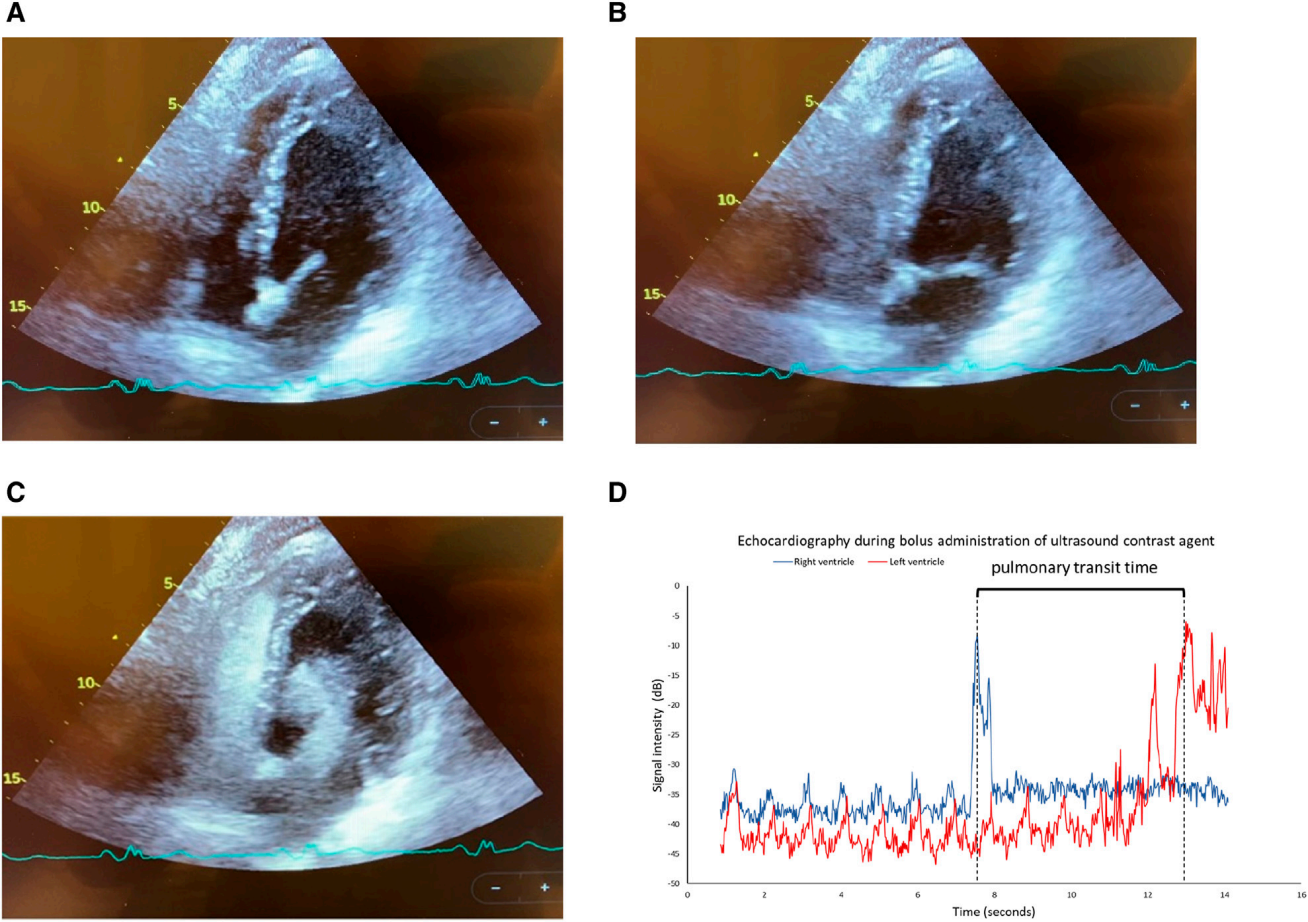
Example of pulmonary transit time determined by echocardiography after 4 min of apnea. RV: Right ventricle. LV: left ventricle. 4D displays ultrasonic determined pulmonary transit time using time-activity curves in RV and LV after a bolus of contrast agent. (A) before contrast; (B) contrast in the RV; (C) contrast in the LV; (D) pulmonary transit time using time-activity curves in the RV and LV.
